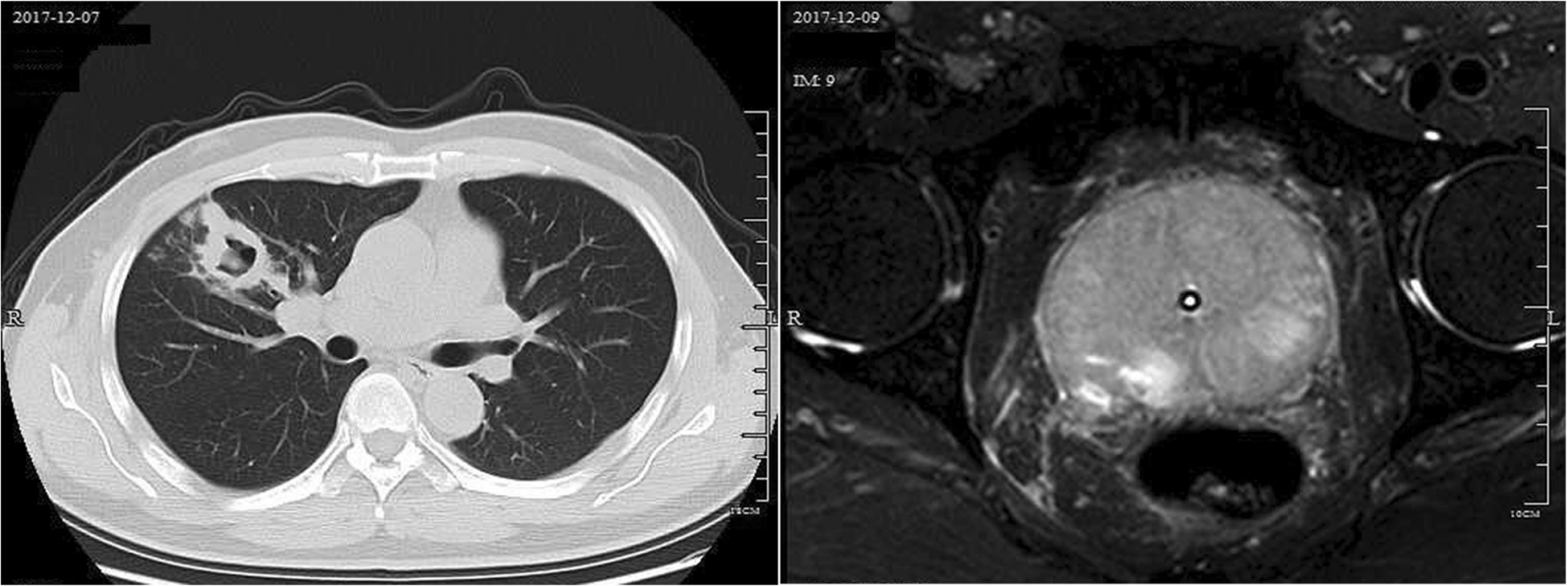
Radiology images of patient. a. Chest CT in Dec 2017 indicated a cavity with effusion in the upper right lung; b. Enhanced MRI of the prostate in Dec 2017 revealed an abnormal patchy signal in the central gland of the prostate
